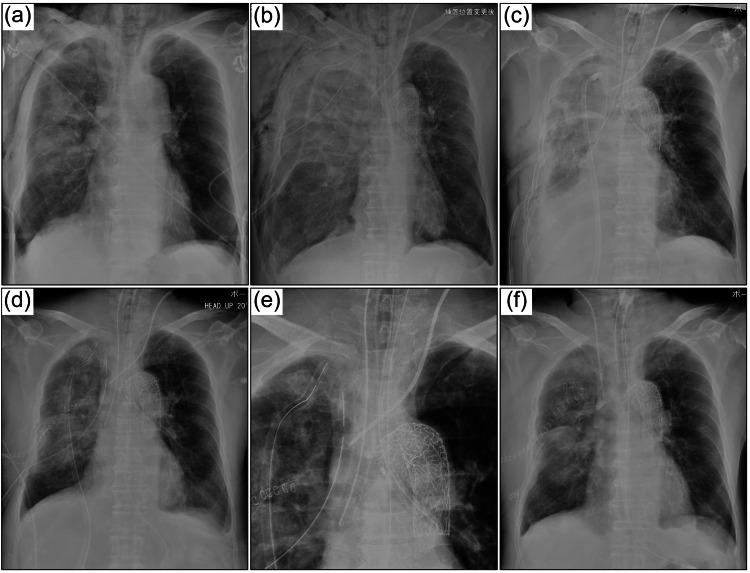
Serial change of chest XP. (a) Admission. (b) After thoracic endovascular aortic repair (TEVAR) and lung resection. (c) Immediately after tracheostomy. (d) Day 2. (e) Expansion of intubation tube location on Day 2. (f) Day 8 (The tracheostomy tube was replaced with a regular tube). XP: X-ray photograph.
